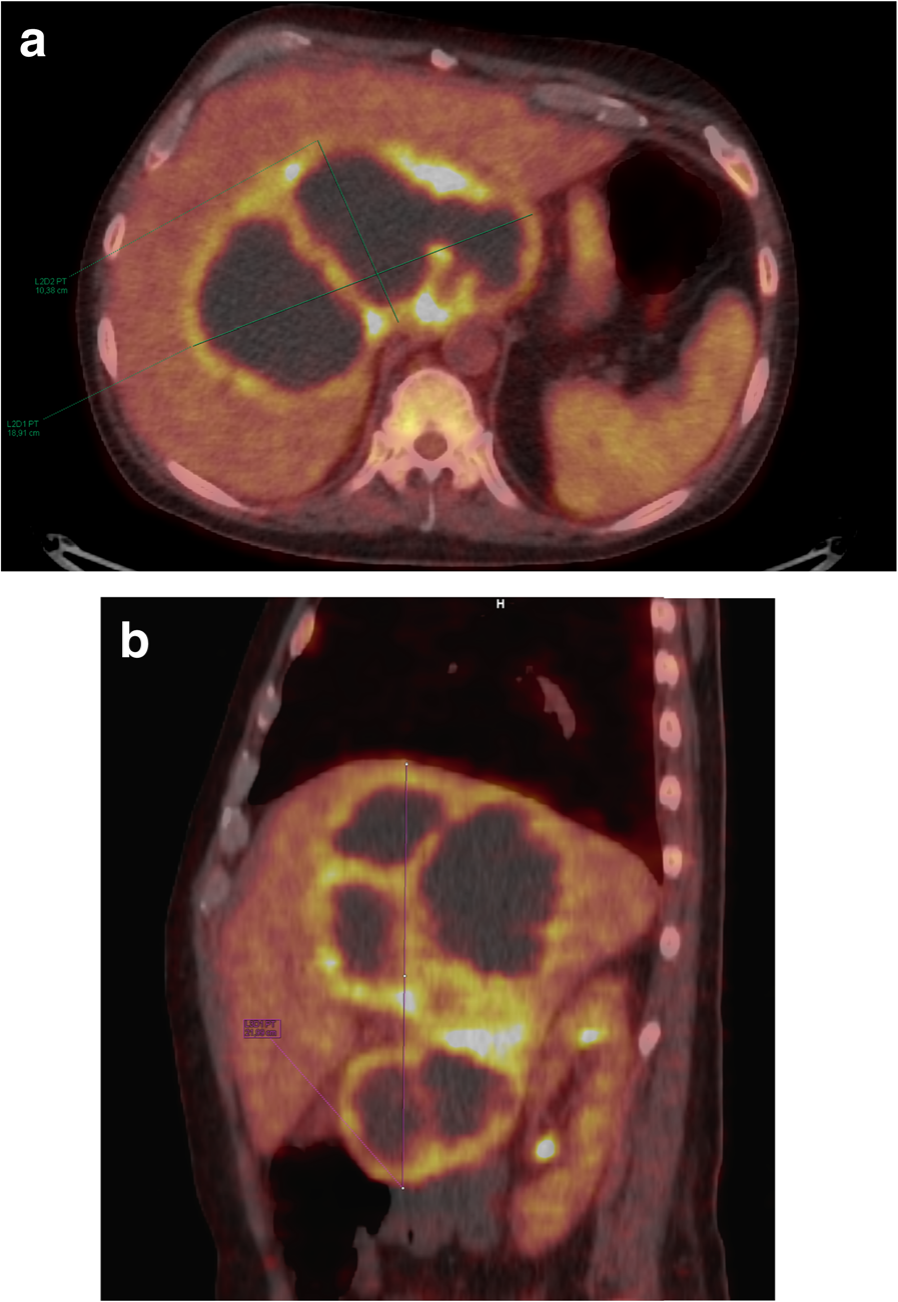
a Transaxial PET-CT scan demonstrating the large multiloculated liver abscess measuring 10.38 × 18.98 cm. b Sagittal PET-CT scan showing the large multiloculated liver abscess measuring 21,99 cm in cranio-caudal dimension
